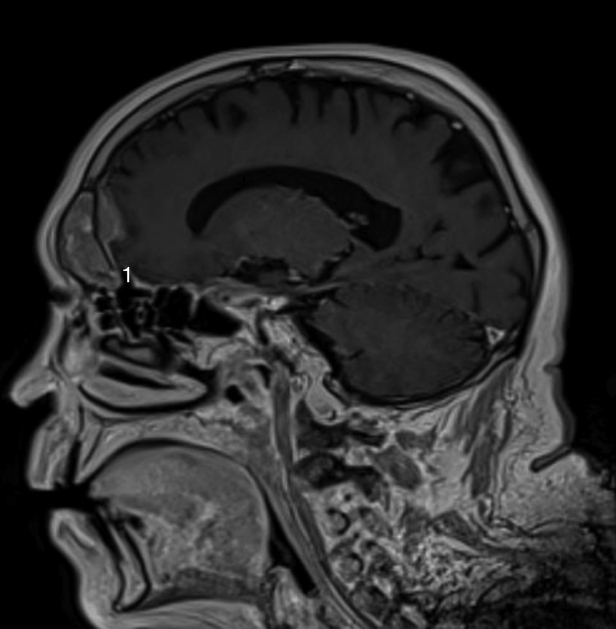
The extension of the sinus frontal metastasis with the loss of the bone hypointense zone showing a dural invasion (MRI, T1).
